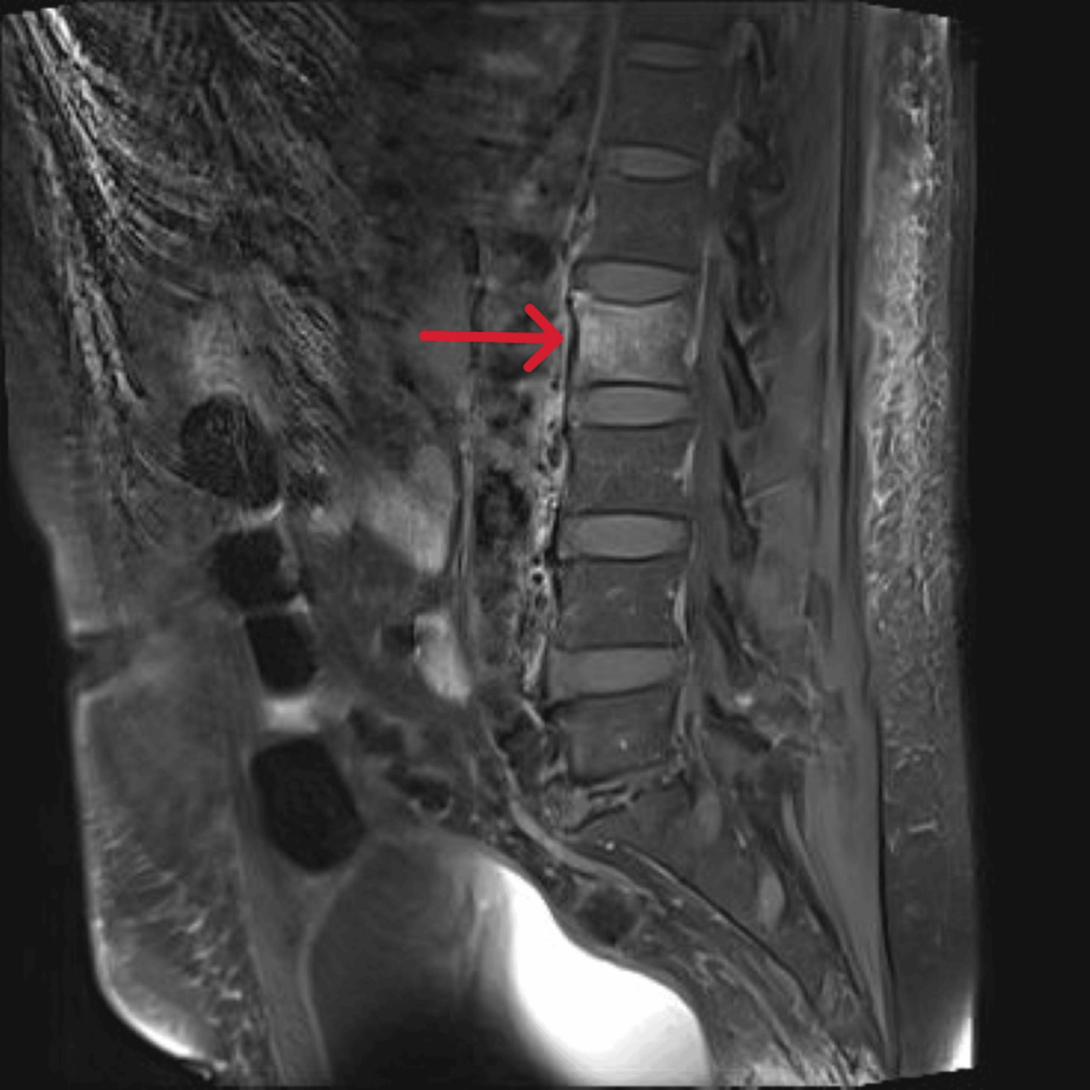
Contrast-enhanced MRI of the lumbosacral spine demonstrating increased signal intensity in the L2 vertebral body, with mild collapse of the superior endplate, consistent with vertebral osteomyelitis The arrow highlights the affected L2 vertebra with marrow changes.
